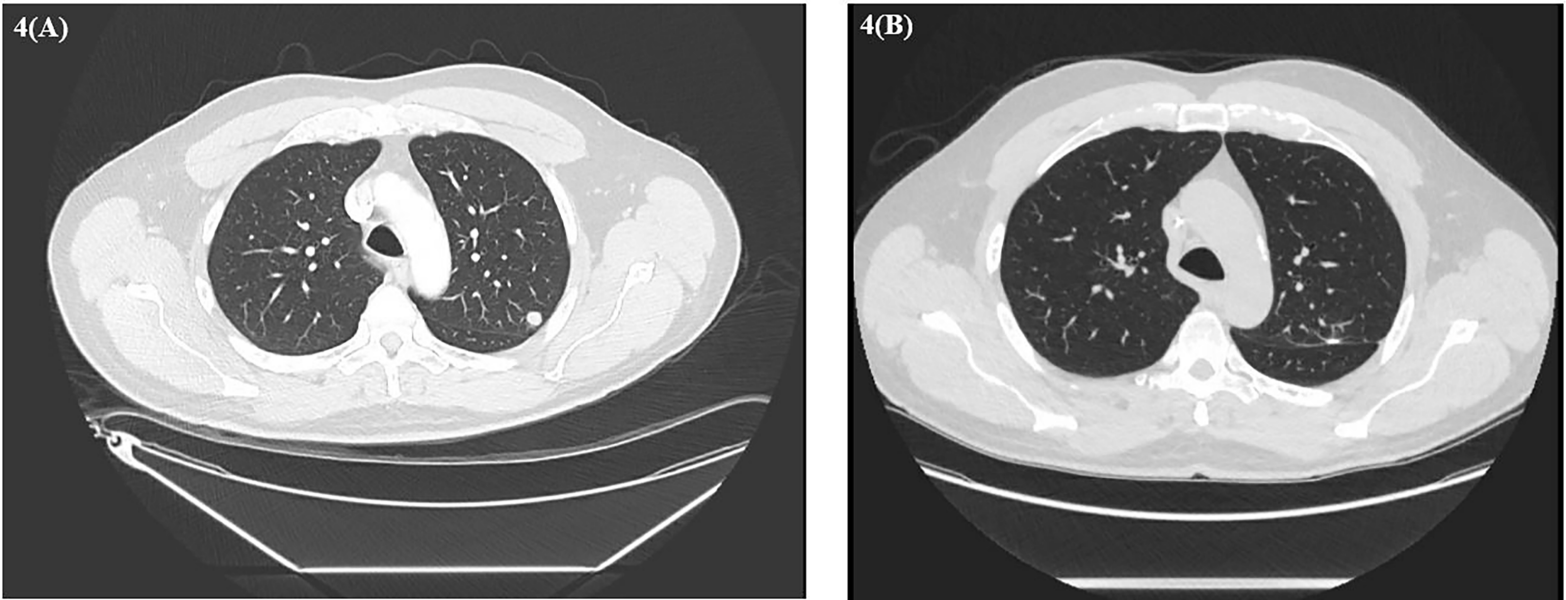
(A) Baseline CT chest demonstrating left upper lobe nodule. (B) CT chest after left thoracoscopic wedge resection.
